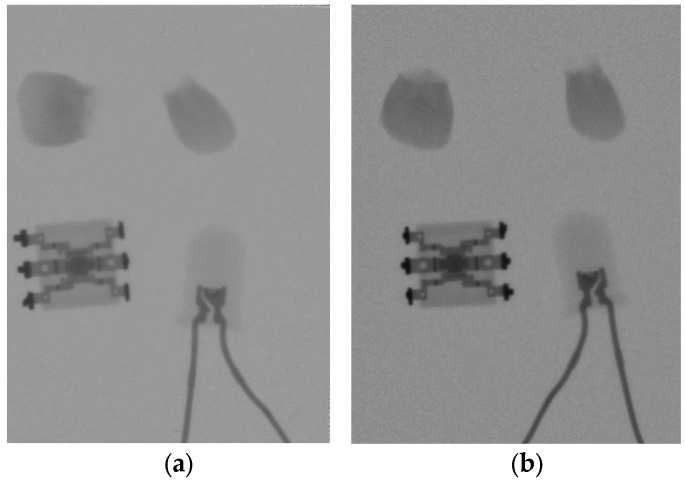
Sample X-ray images for comparison: (a) resin screen 34 mg/cm^2^; (b) Min-R 2190. Acquisition parameters: 70 kVp; 63 mA; 1 s; 20 mm Al filter; offset and gain correction. Setting: teeth, optocoupler, LED. The dimensions of the X-ray images are 1200 × 1600 pixels, corresponding to an active area of 27 × 36 mm^2^ [12].
